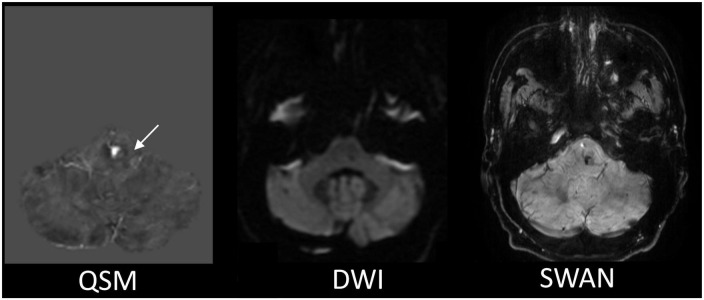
The representative MR images comparison for the CCM detection. The QSM displays the most apparent boundary of the CCM from among all the MR images we acquired. The white arrow is pointing to the location of the CCM.
